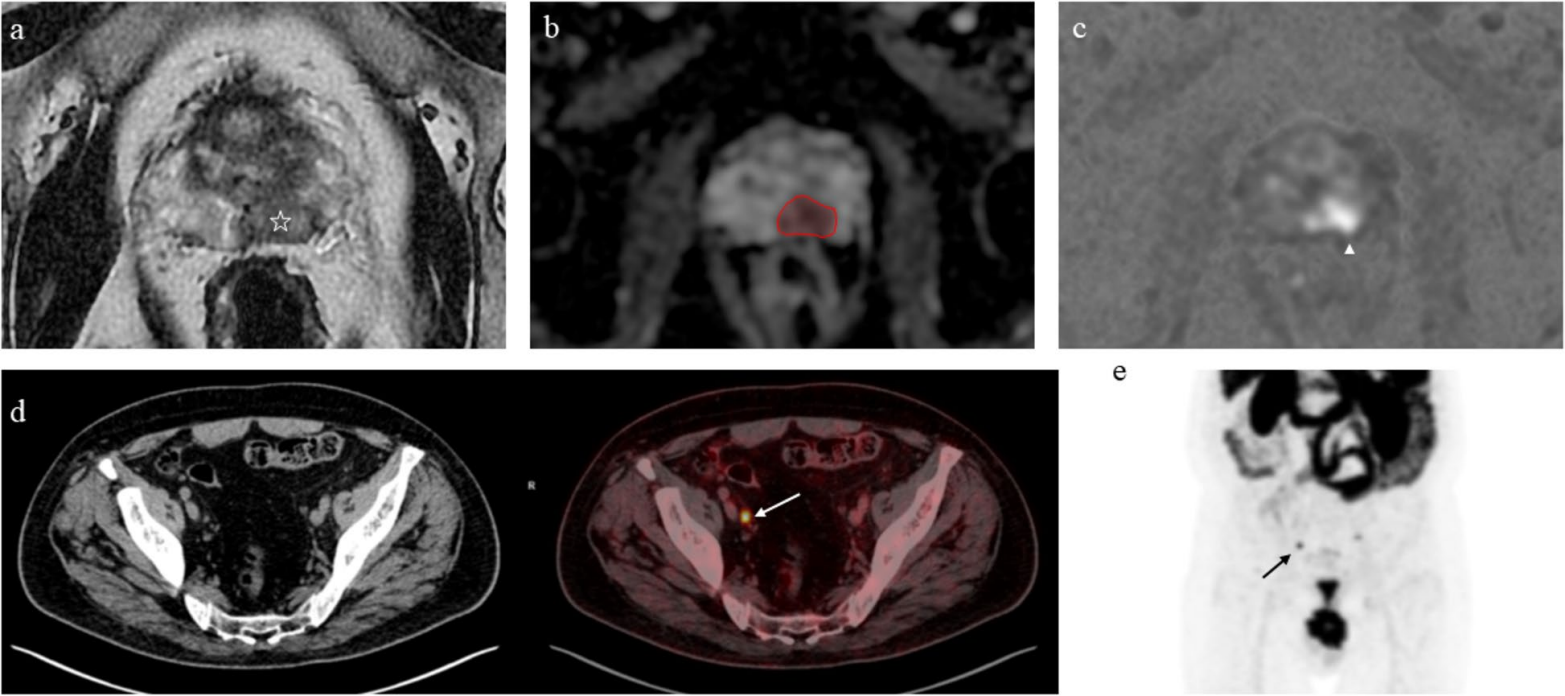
Seventy-two-year-old patient who underwent radical prostatectomy for prostate cancer (at pathology, Gleason score 3 + 4). Staging MRI shows a large peripheral zone (left posteromedial) lesion with intermediate signal on T2-weighted images (a, lesion epicentre marked with white star), focal and marked hypointensity on the ADC map (b, red region of interest) and corresponding hyperintensity on high *b* value DWI (c, white arrowhead). Three years after treatment, rise of PSA value determines biochemical recurrence. [^68^Ga]Ga-PSMA-11 PET/CT (d and e) shows a small but concerning right external iliac lymph node. *ADC* Apparent diffusion coefficient, *DWI* Diffusion-weighted imaging, *MRI* Magnetic resonance imaging, *PET/TC* Positron emission tomography/computed tomography, *PMSA* Prostate-specific membrane antigen, *PSA* Prostate-specific antigen
